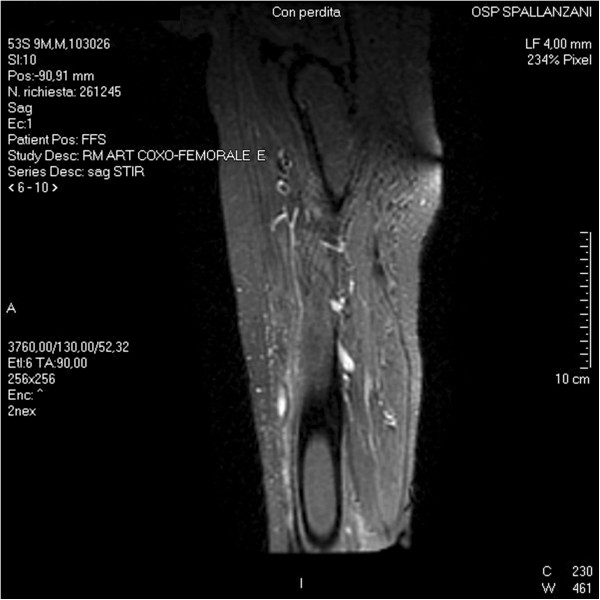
Magnetic Resonance Imaging performed after a month of antibiotic therapy shows complete resolution of the phlegmon.
